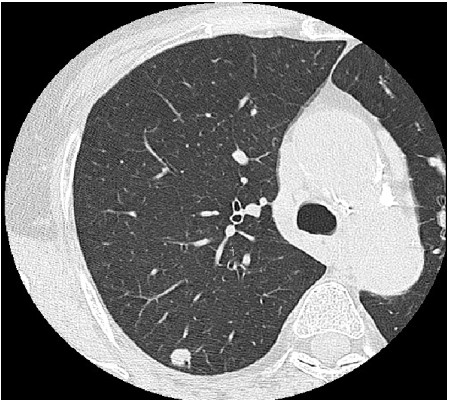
CT image. A nodular shadow, 10 mm in diameter, is seen in the right S10 region.
